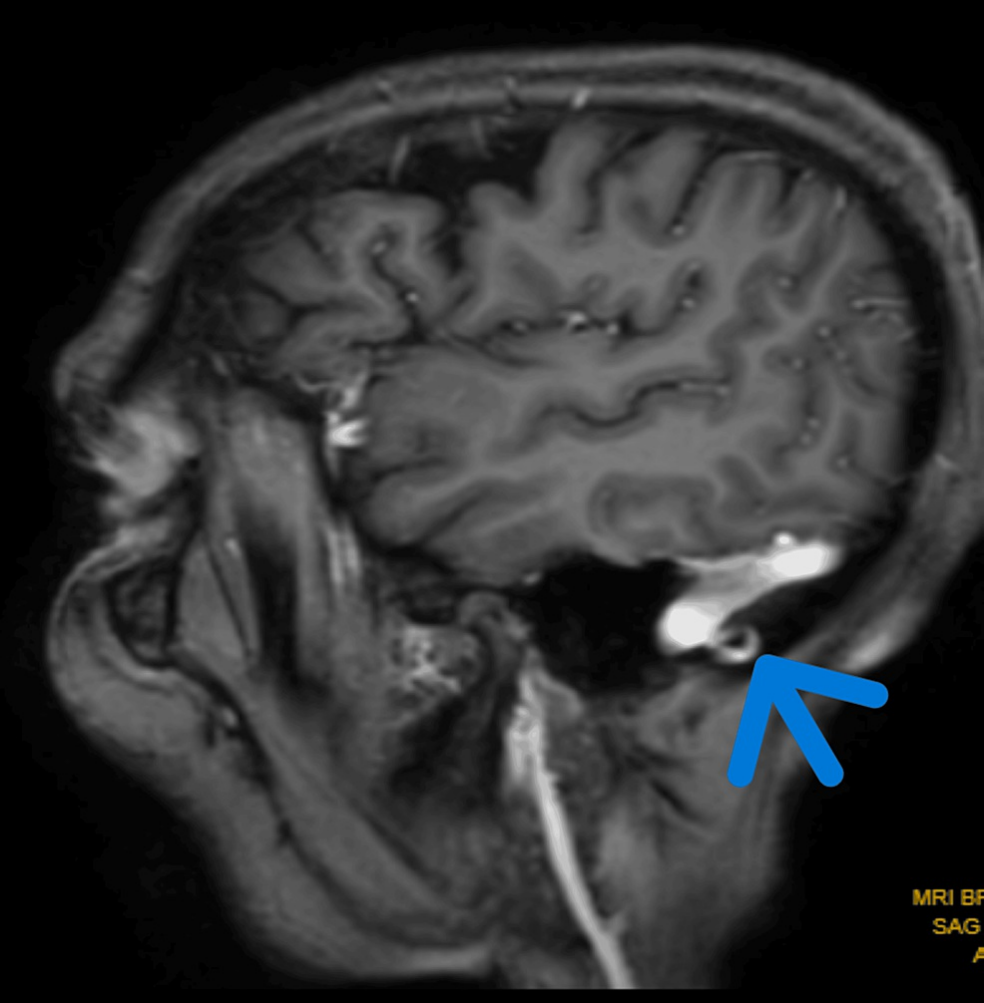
MRI of the brain, which revealed altered signal intensity lesions in both halves of the pons and left middle cerebellar peduncle MRI: magnetic resonance imaging
